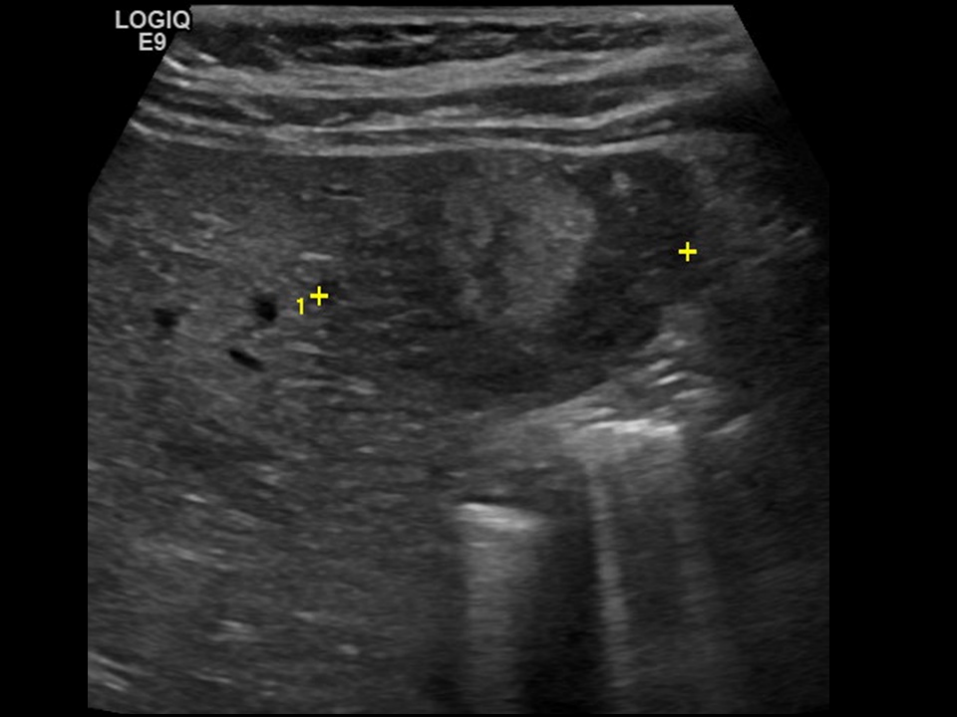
Transverse Ultrasound Image of the Liver Transverse ultrasound image of the liver demonstrating a 3 centimeter heterogeneous lesion in the left inferior liver edge (indicated by measurement calipers). This particular lesion retrospectively corresponded with the area of subtle T2 hyperintensity on initial whole body MRI and is also the lesion that would ultimately get resected (with pathology confirming focal nodular hyperplasia). Several additional similar-appearing lesions were also detected on this ultrasound study (not included in this image).
